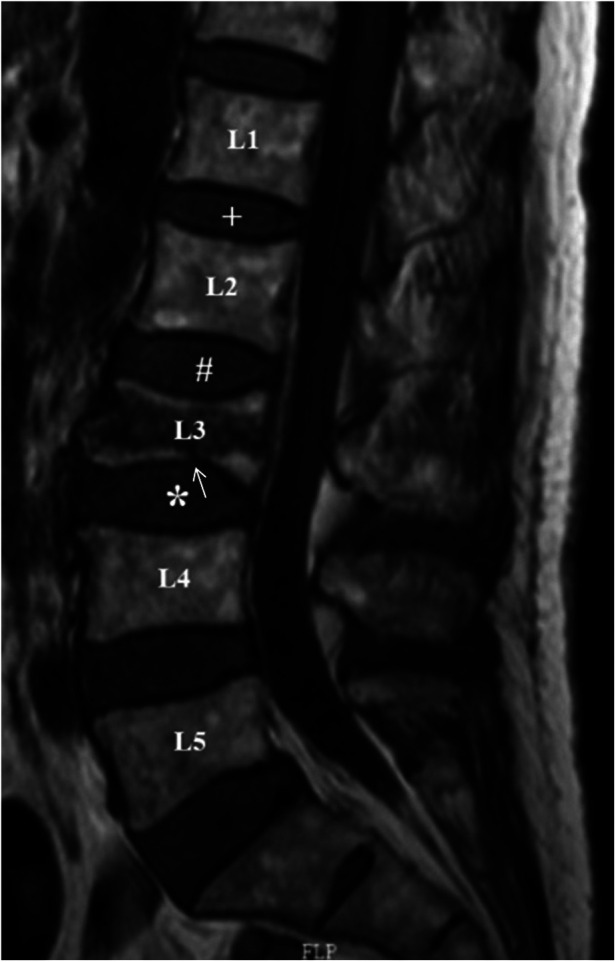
Definition of cranial, caudal and control discs. In the mid-sagittal view of MRI T1WI, L3 is the fractured vertebral body. L2/3 disc (#) and L3/4 disc (*) were defined as the cranial and caudal adjacent discs, respectively, and L1/2 disc (+) was defined as the internal control disc. The white arrow indicates caudal endplate injury of L3.
